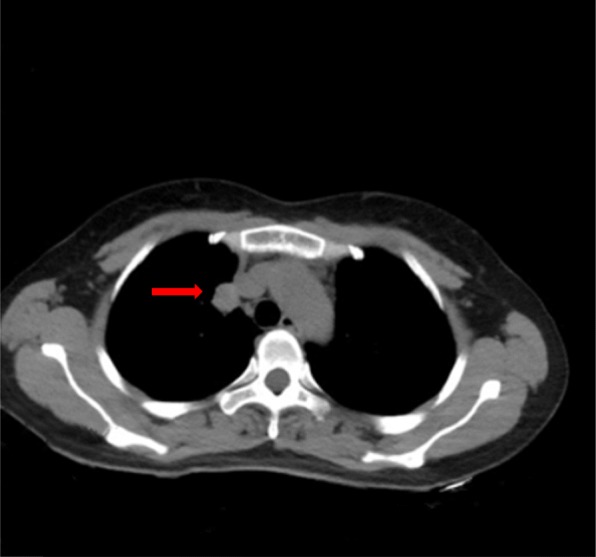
Chest computed tomography showed a 2.1 × 1.7 cm well-defined round mass exhibiting mild, heterogeneous internal enhancement at the periphery of the right superior lobe
